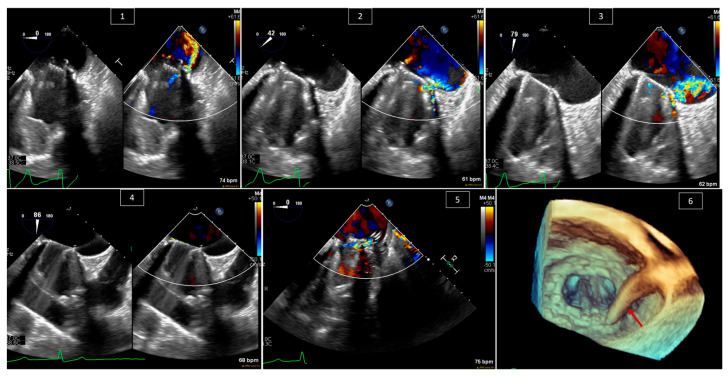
Paravalve leak plugging in a 51-year-old male with symptomatic paravalve leak. (1–3) Looking at the posterior paravalve area and the leak at different angles, with and without color Doppler—0 degrees in (1), 42 degrees in (2), and 79 degrees in (3). The intervention. (4) Guiding the wire across the paravalve leak and confirming its position with and without color Doppler. (5) Seeing the catheter inside the paravalve leak area with color Doppler. (6) The catheter (red arrow) in the paravalve leak area in 3D.
